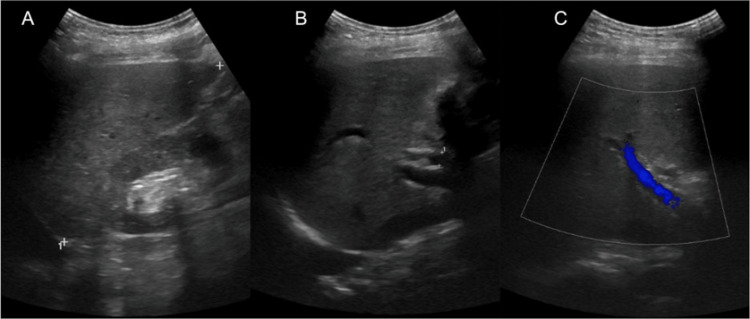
Ultrasound of the liver. (A) Echogenic liver with normal contour, measuring 12 cm. (B) The common bile duct measuring 7 mm. (C) A patent portal vein.
